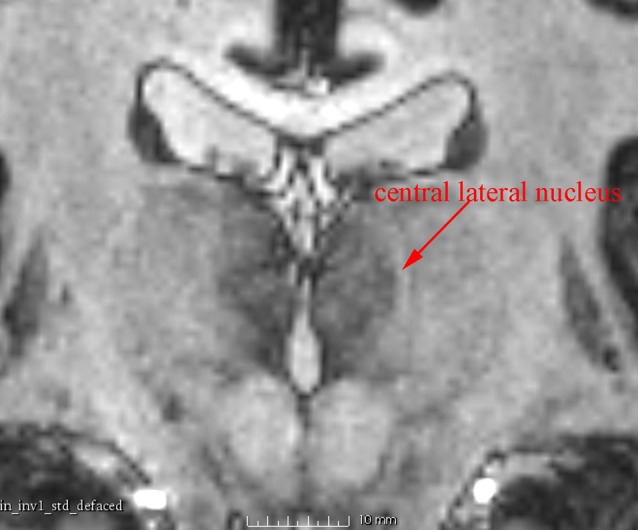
A coronal 7 T MR image showing the central lateral nucleus, a structure about 1 mm wide, which is clearly identified as a high-intensity zone.
